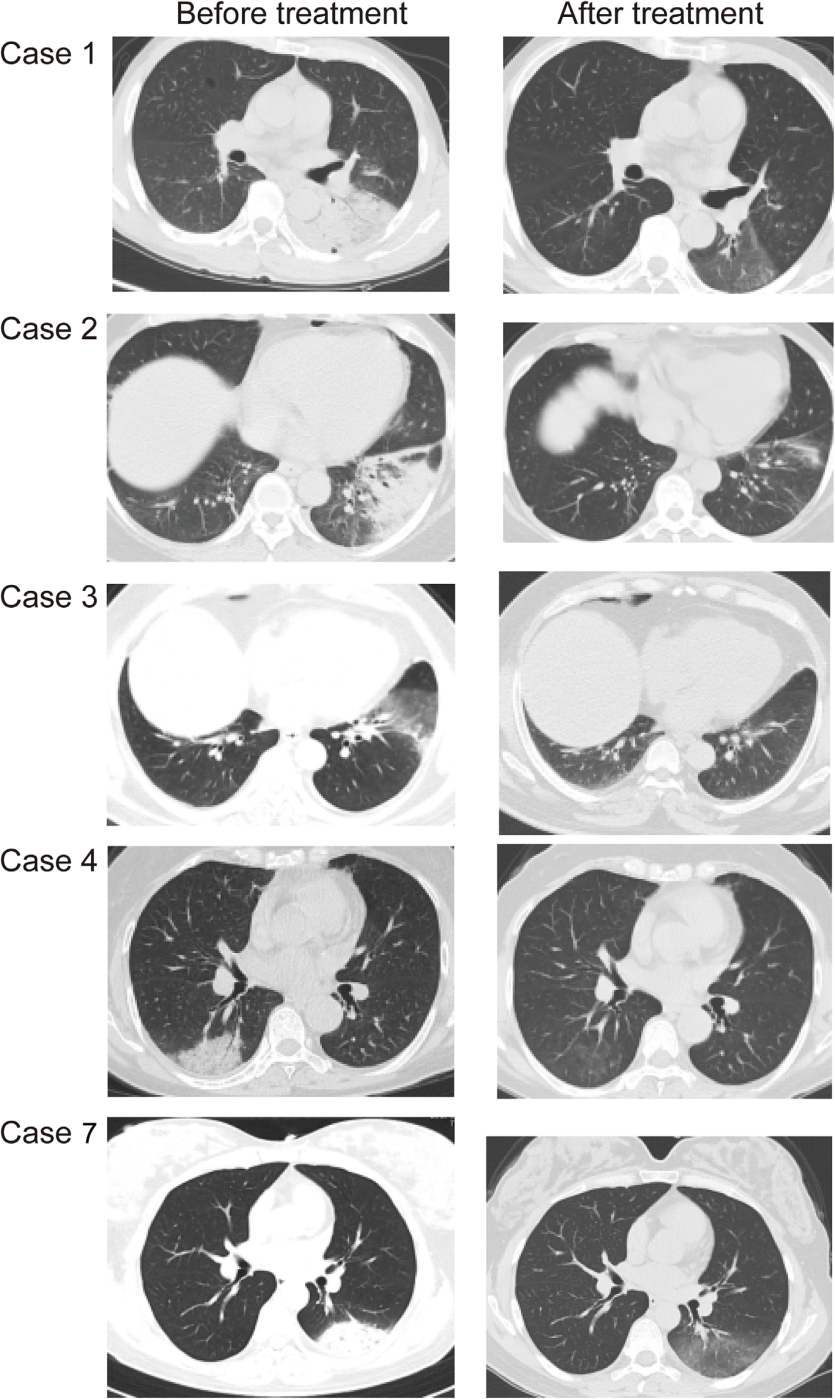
Pulmonary CT findings among the five patients before and after treatment.
